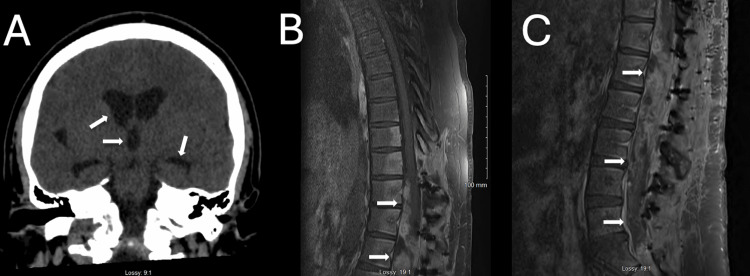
(A) Postoperative computed tomography head without contrast shows stable lateral ventricles, third ventricle, and temporal horns (white arrows). (B) Postoperative magnetic resonance imaging thoracic and lumbar spine with contrast show postoperative changes and residual tumor (white arrows).
